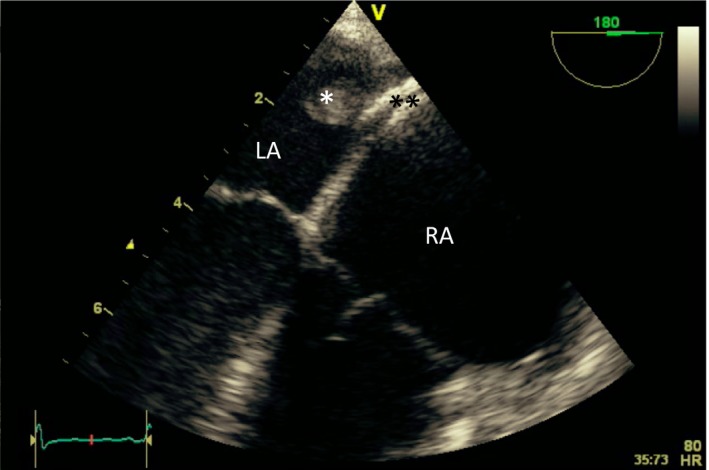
Transesophageal echocardiogram (midesophageal at 180 degrees) showing a thrombus (*) attached to the left atrial disc of an Amplatzer PFO occluder (). LA indicates left atrium; RA, right atrium.
